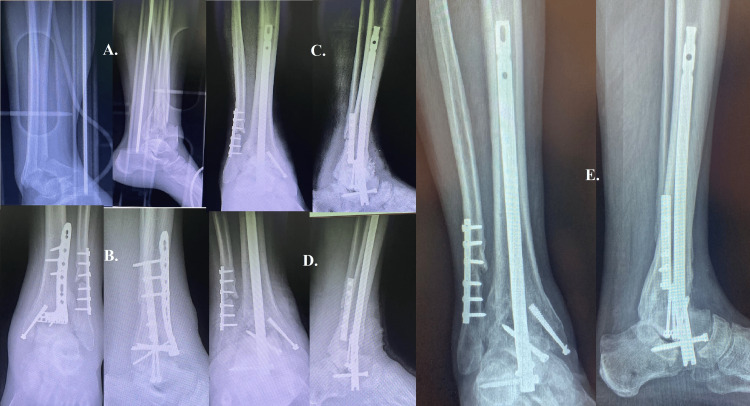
Case seven A: shows the comminuted fracture, which was hard to spot due to the patient's restlessness. B: shows fracture reduction and fixation three weeks after the fracture, with fibrosis preventing precise anatomical alignment. C: shows the postoperative radiograph two years later with a centromedullary rod with a bone substitute used to treat tibia-calcaneal-talar arthrosis. D: shows arthrodesis one month after intervention. E: successful arthrodesis one year after surgery, with the patient walking almost normally.
